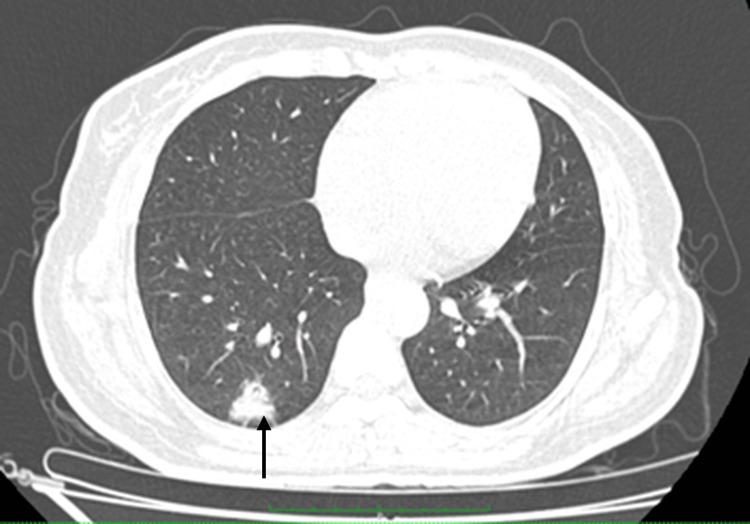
CECT axial section showing an enhancing nodule with spiculated margins (arrow) noted in the posterobasal segment of the right lower lobe, suspicious of a malignant nodule. CECT: contrast-enhanced computed tomography
